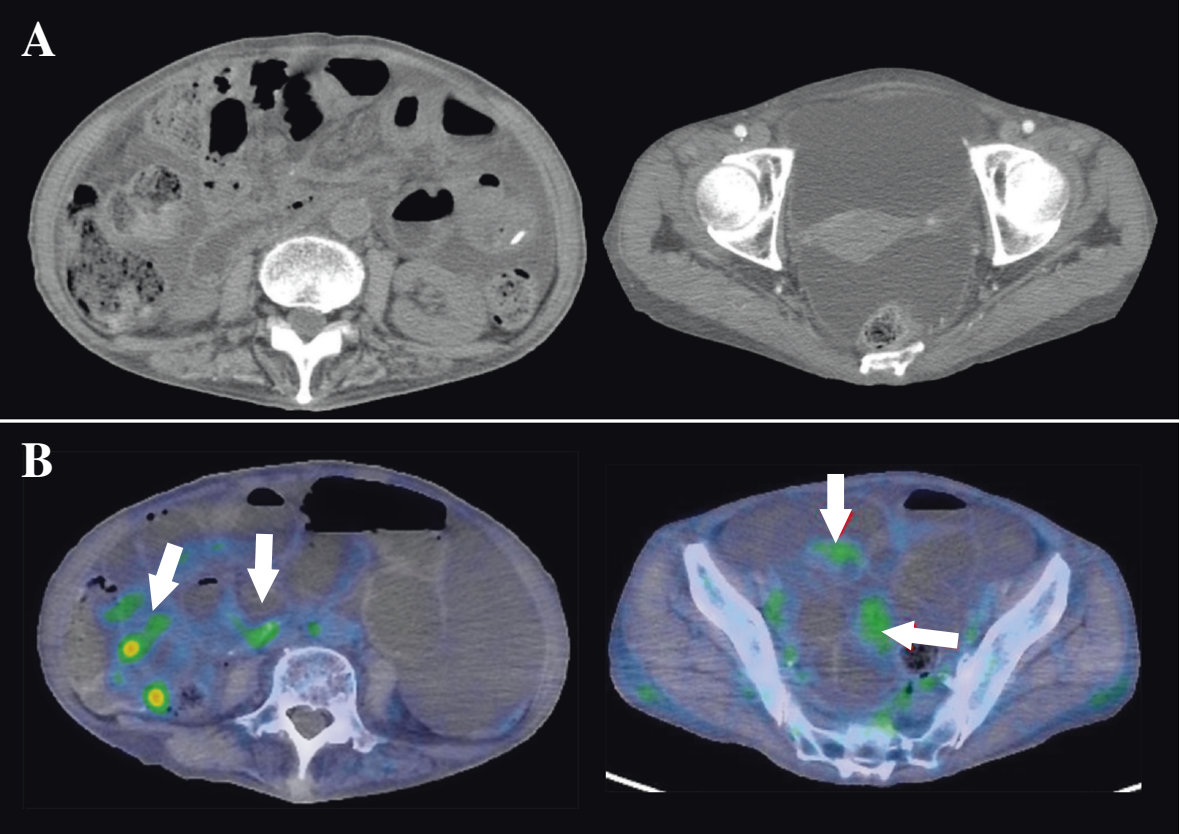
Increase in ascites and diffuse low-grade FDG uptake. Abdominal CT shows an increase in ascites and mild thickness of the small intestinal wall (A). Diffuse low-grade FDG uptake along the small intestinal wall (white arrows) (B).
